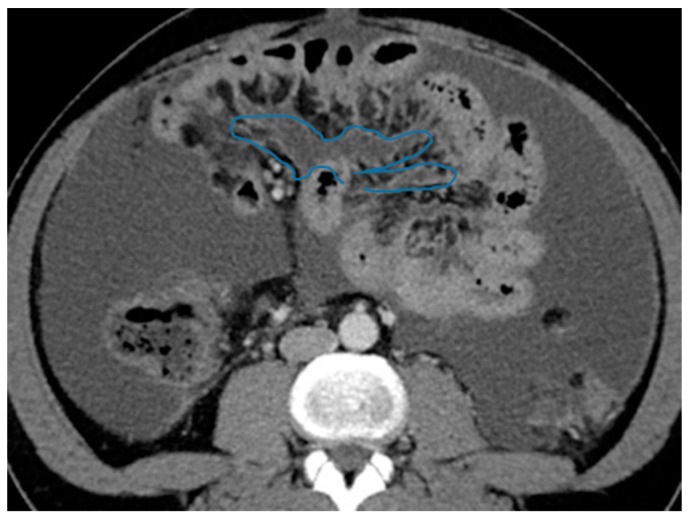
Axial CE-CT. PC from colon adenocarcinoma: involvement of the mesenteric leaves (note the nodular thickening and enhancement, highlighted in blue) that becomes more apparent with ascites.
